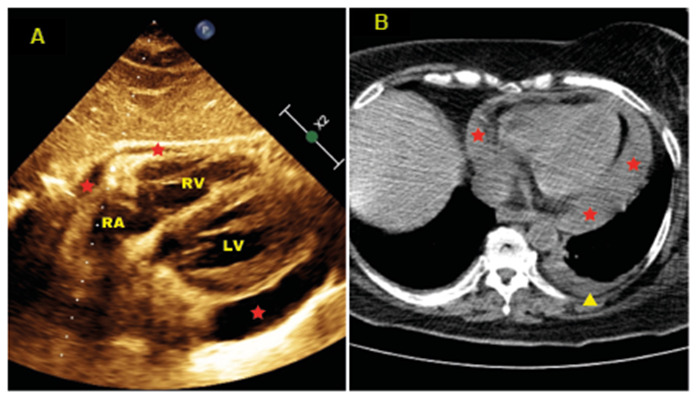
(A) - Bedside echocardiographic image (long parasternal axis view) showing signs of pericardial effusion (red *). (B) - Chest computed tomography showing circumferential pericardial effusion (red *) and mild left pleural effusion (yellow arrowhead). RA: right atrium; RV: right ventricle; LV: left ventricle.
